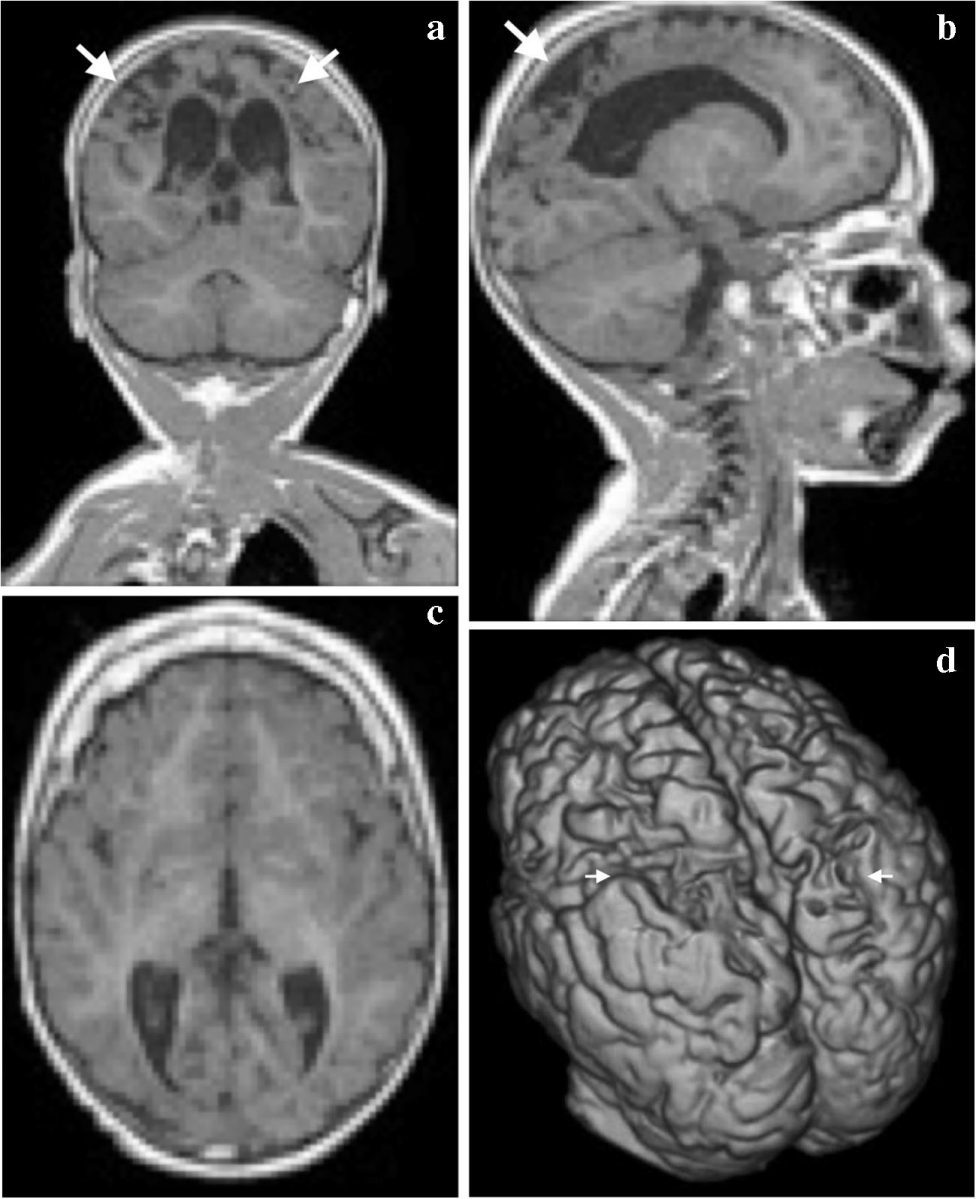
A 2-year-and-2-month-old boy who suffered an acute-profound perinatal hypoxic ischemic injury. a–c Multiplanar coronal (a), sagittal (b) and axial (c) reconstructions of T1-weighted magnetic resonance images demonstrate bilateral, relatively symmetric peri-Rolandic volume loss (*arrows *in a and b). d High-fidelity three-dimensional surface mesh achieved by generating a standard tesselation language model using the Statistical Parametric Mapping version 12 technique accurately demonstrates the fine cortical detail at the atrophic peri-Rolandic regions (*arrows*)
